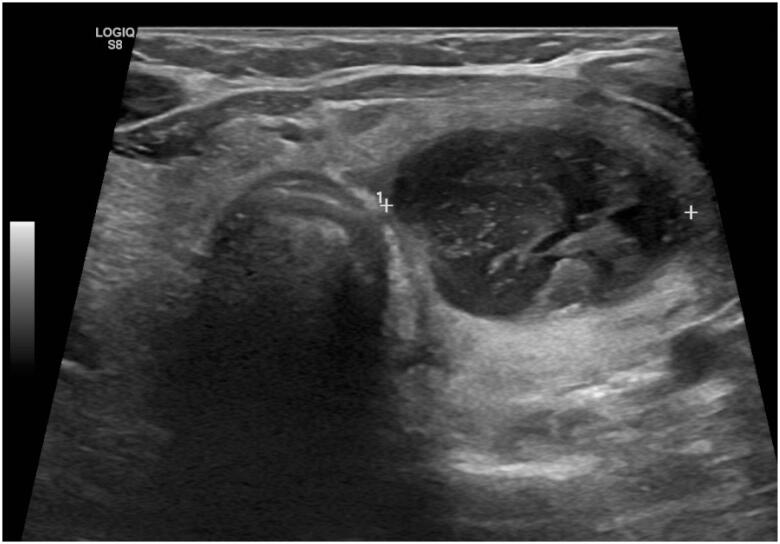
First thyroid ultrasonography performed showing a nodular lesion at left thyroid lobe with a solid central component, well delimited, without hypervascularity measuring 36 × 36 mm.
